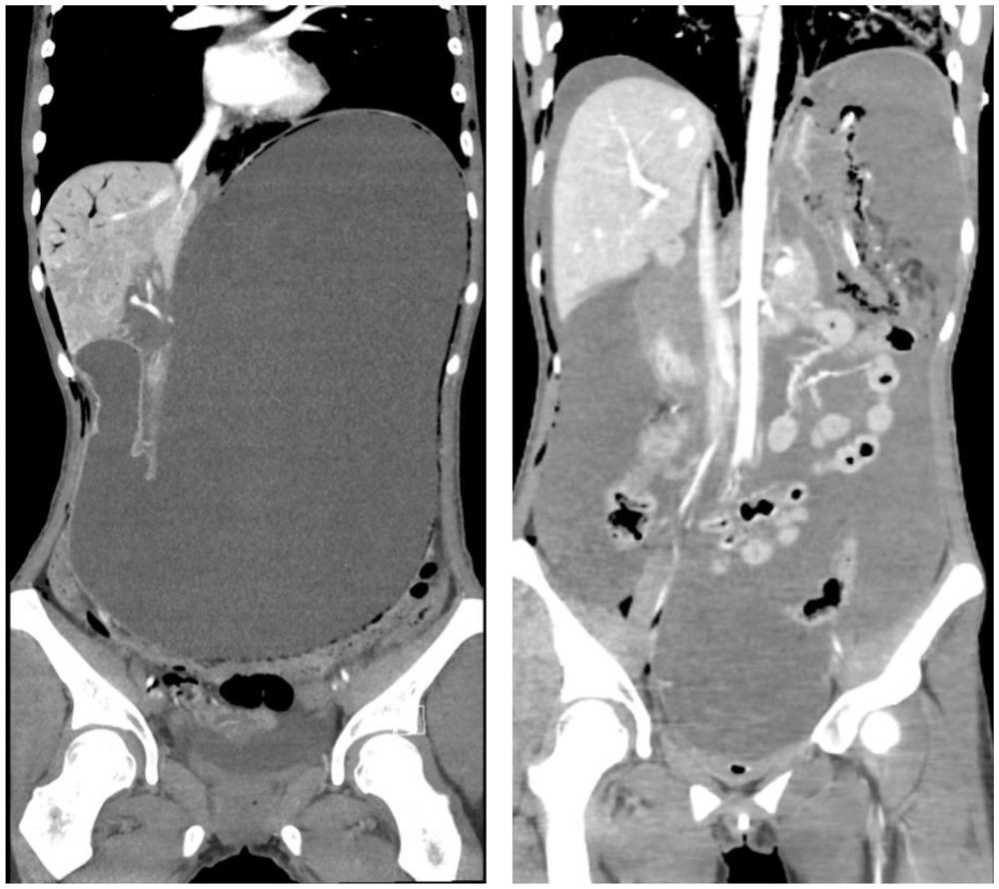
Coronal reconstruction. Left shows the initial CT, pre-perforation, showing pneumatosis the wall of the gastric fornix, adjacent to the lung, and in the gastric body, projecting within in the pelvis. On the right, the second CT is shown, acquired after deterioration with a collapsed stomach after perforation and pneumatosis in the gastric fornix.
